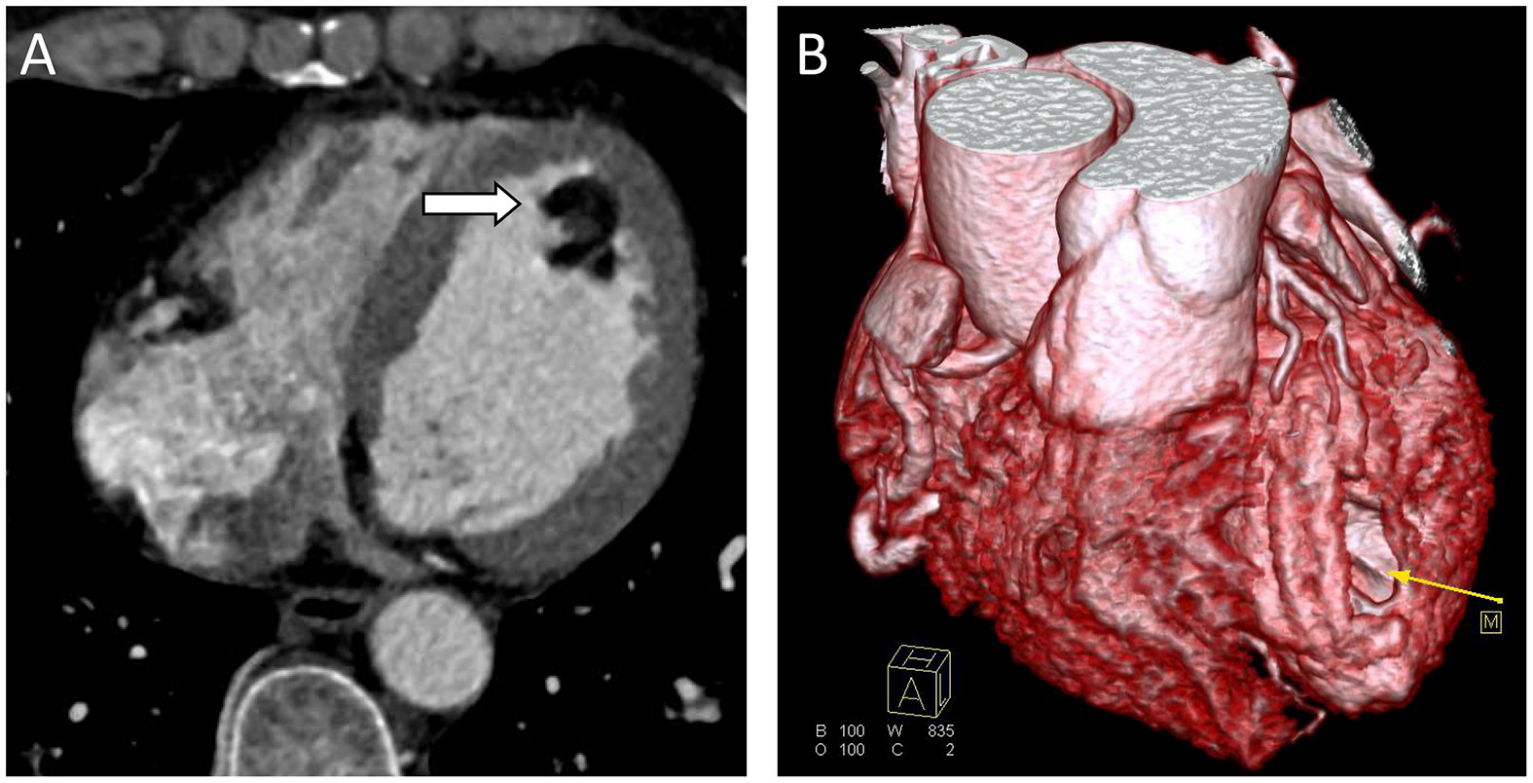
Computed Tomography (CT) scans. (A) CT scan showing a well-defined hypodense tumor in the left ventricle (arrow). (B) CTA 3D reconstruction; hypodense tumor (yellow arrow).
